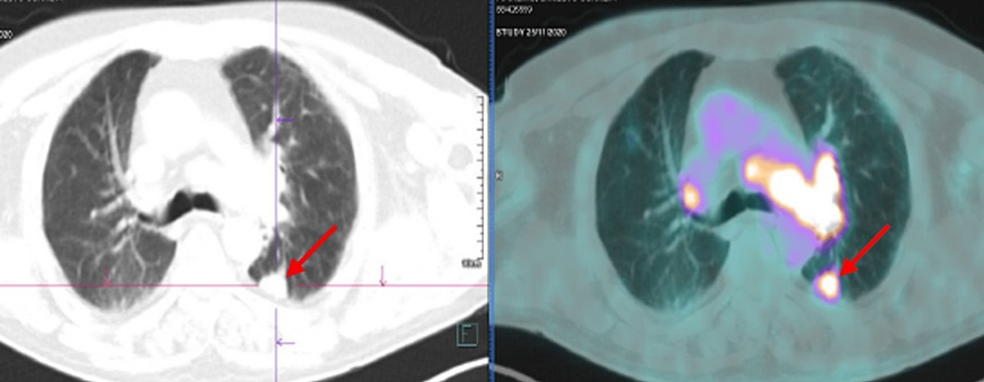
PET-CT scan showing a mass in the superior segment of the inferior lobe of the left lung (arrows) and left hilar lymphadenopathies/conglomerates. PET: positron emission tomography; CT: computed tomography
